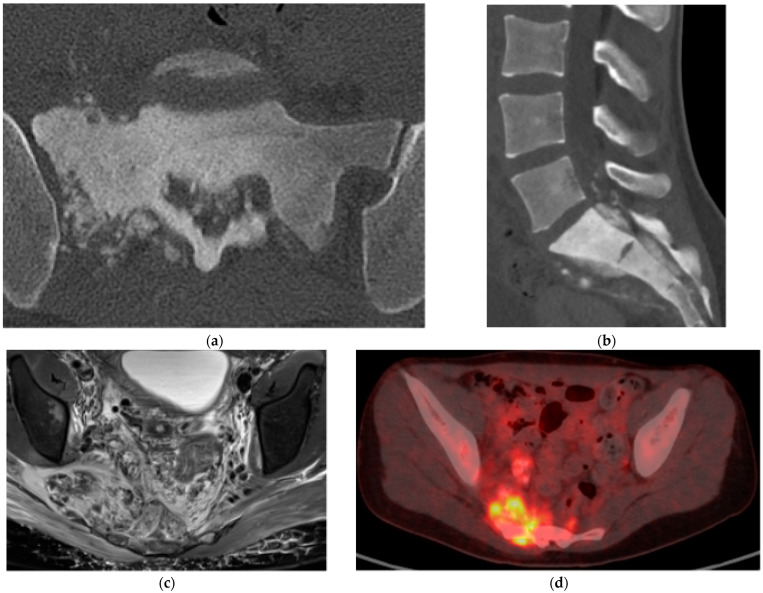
Osteosarcoma. (a) Axial CT bone window, aggressive, sclerotic lesion centered in the right sacral ala with new bone formation (osteoid matrix). (b) Sagittal CT bone window, osteoid matrix in the sacral canal and in the presacral space. (c) Axial T2 MR, large soft tissue mass extending into the pelvis. Multiple low-signal-intensity nodules compatible with osteoid matrix. (d) FDG PET/CT, increased uptake in the mass representing increased metabolic activity.
